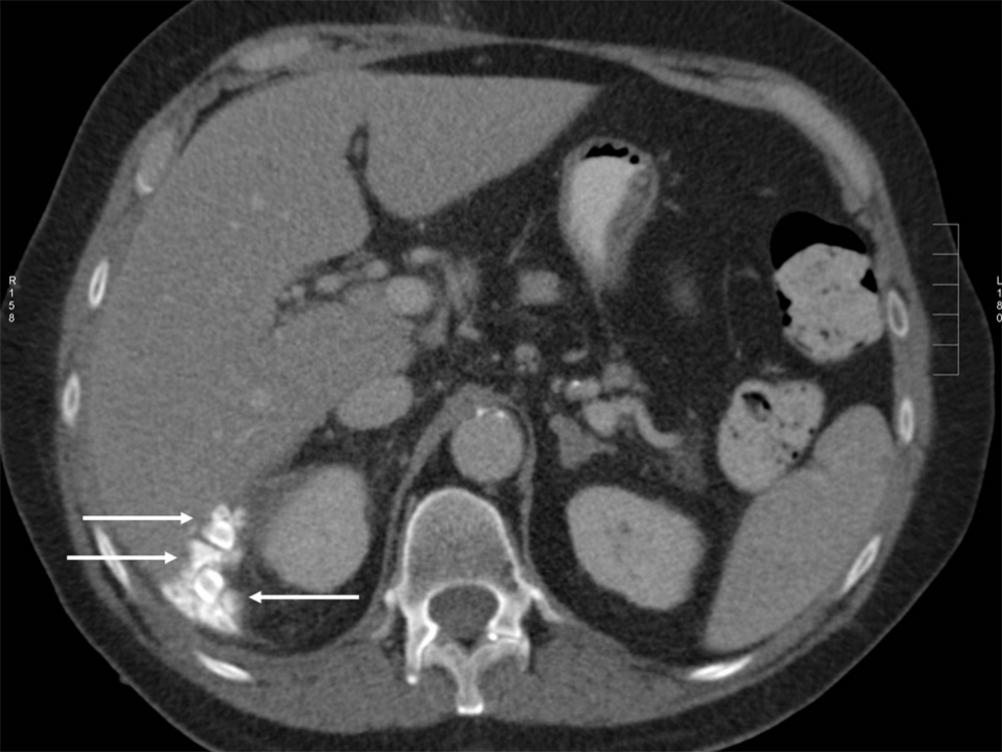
Axial contrast-enhanced CT scan of the abdomen demonstrating multiple spilt gallstones within Morison's pouch (arrows).
